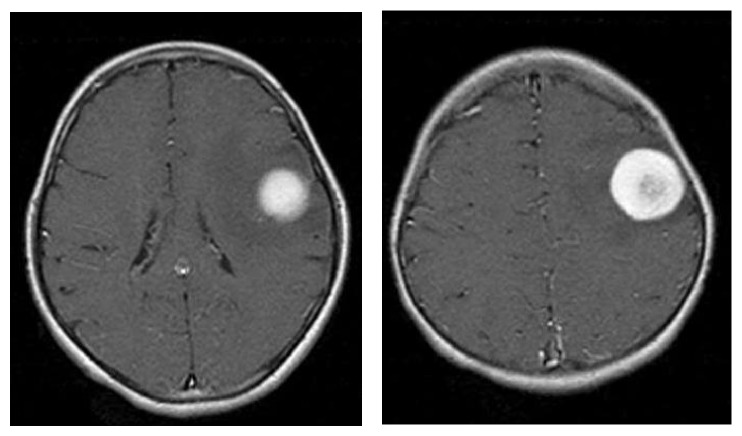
Sample input MRI brain images.
